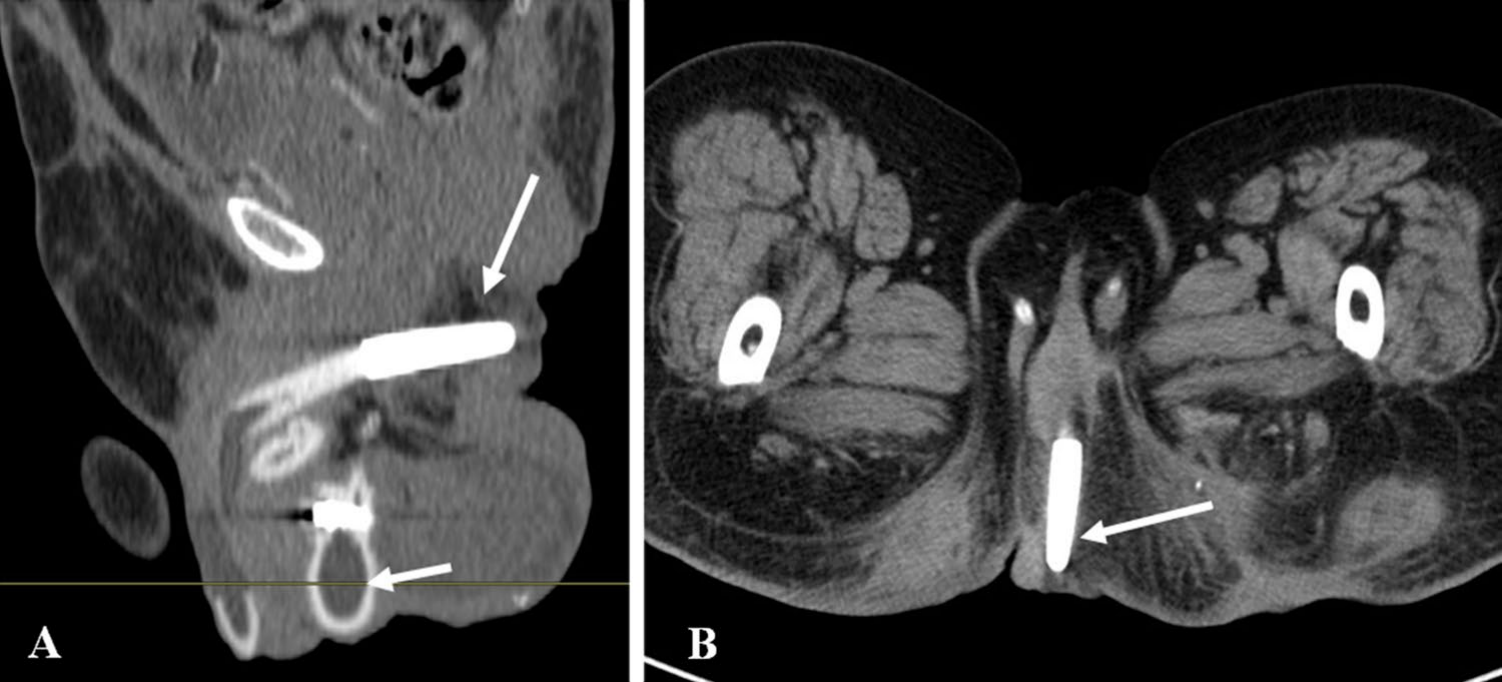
75-year-old man with penile prostheses inserted 10 years back after radical prostatectomy. Sagittal (a) and axial (b) CT images show the significant posterior migration of one of the cylinders (arrow) reaching the intergluteal cleft. Scrotal pump also seen (short arrow)
